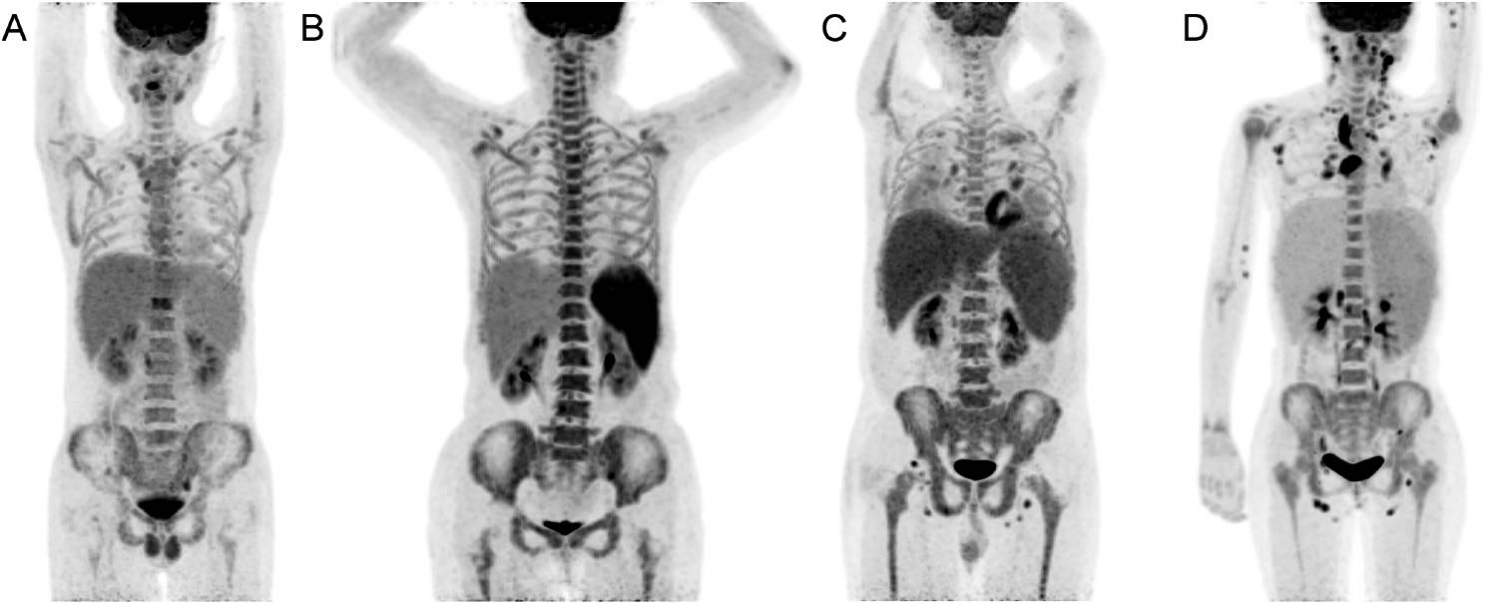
^18^F-FDG PET/CT maximum intensity projection images depicting four HLH patients with EBV infection, wherein the combined model effectively differentiated the cause. Focal hypermetabolic lesions of bone were observed in a 19-year-old male (A) and a 64-year-old female (B), but no lymphoma was found by bone puncture or lymph node biopsy. A 53-year-old male (C) and an 18-year-old female (D) showed only hypermetabolic lymph nodes with symmetrical distribution and were diagnosed with Hodgkin lymphoma and NK/T-cell lymphoma, respectively, based on lymph node biopsy
